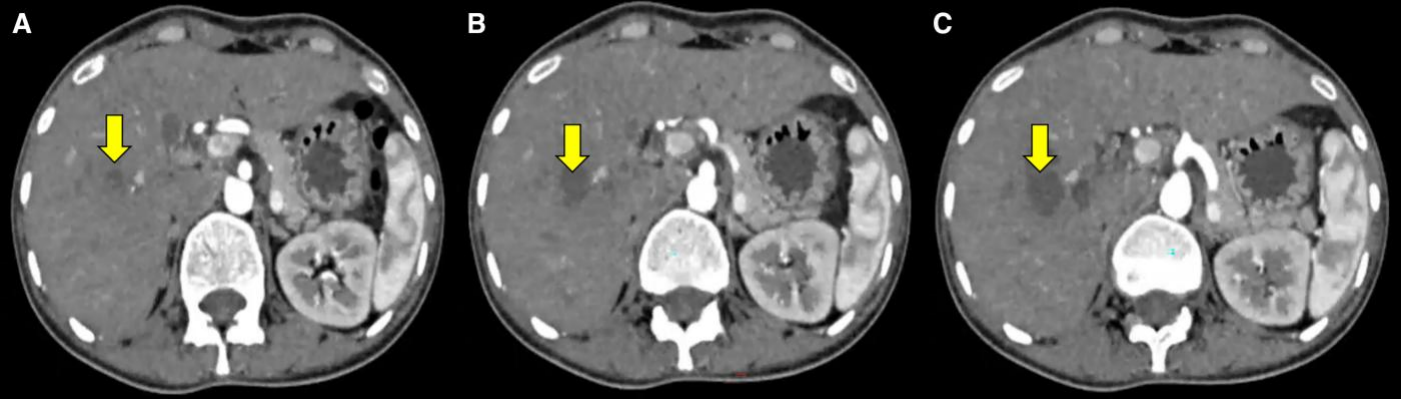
(A–C) Computed tomography (CT) of abdomen at 6 weeks posttreatment showing remarkable improvement with resolving small lesions in right lobe of liver (arrows).
